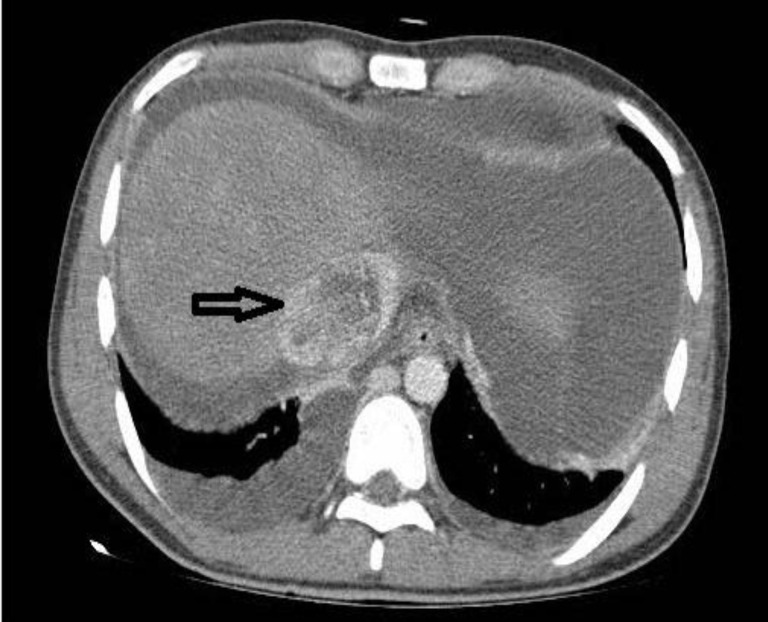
Contrast-enhanced abdominal computed tomography showed extension of the mass to IVC (black arrow) and ascitis.
